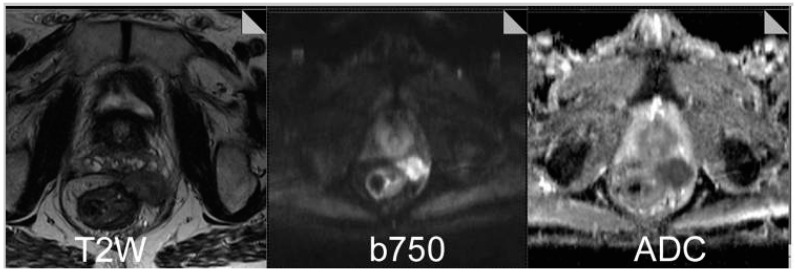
Restricted diffusion within rectal cancer with extension into the perirectal space. T2-weighted image demonstrate a well-circumscribed lesion in the perirectal space. Diffusion-weighted image obtained at a b value of 750 demonstrates a high signal, and corresponding ADC map demonstrates relatively restricted diffusion within the tumour. Figure reproduced with permission from Padhani et al. [24].
